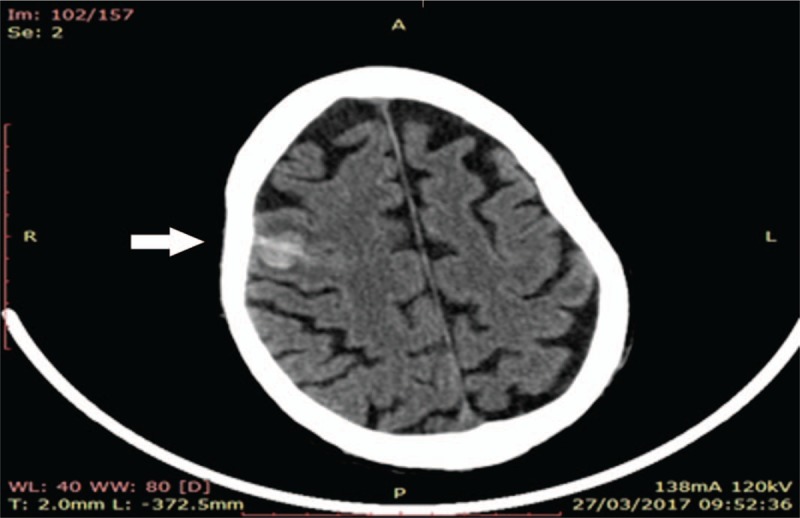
A brain CT reveals focal hemorrhage of 15 mm at the cortico-subcortical right frontal site.
